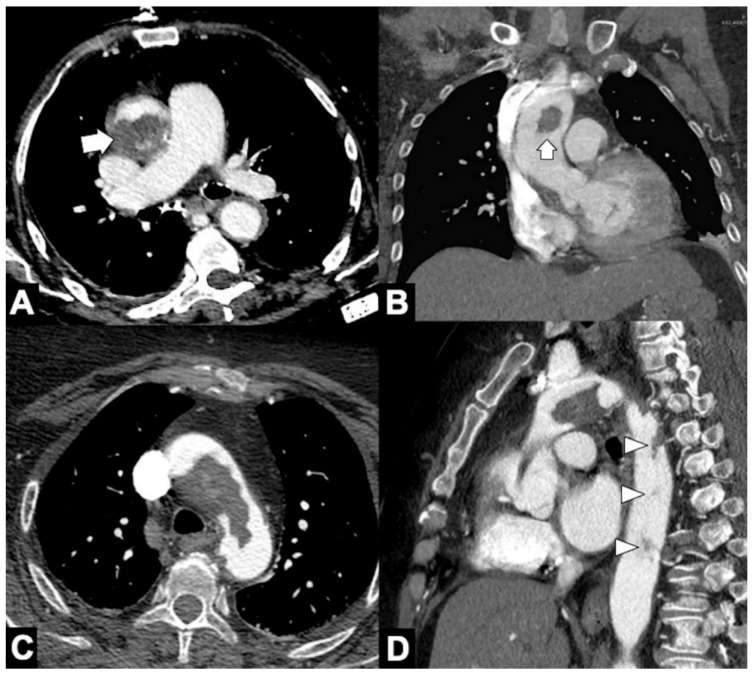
CTA of acute diffuse aortic atheromatous embolization in a 46-year-old COVID-19 pneumonia patient with acute cerebral vascular event. (A) CTA axial scan shows extensive and irregular atheroma of the ascending aorta (arrow). (B) Coronal MIP reconstruction image confirms ascending aorta filling defect (arrow). (C) In the same patient, CTA axial scan shows extensive irregular atheroma of the transverse aortic arch. (D) Sagittal MIP reconstruction image confirms extensive arch atheroma and friable floating thrombi in descending thoracic aorta (arrowheads).
